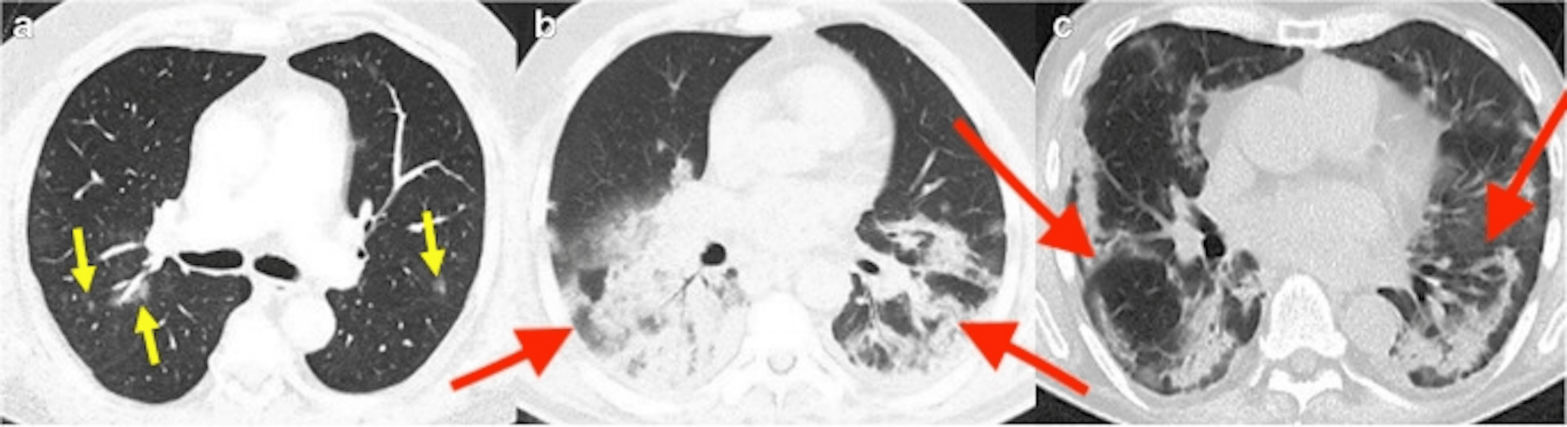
Representative CT findings in COVID-19 pneumonia CT: computed tomography Transverse CT images show (A) ill-defined ground-glass nodules bilaterally (yellow arrows), (B) extensive ill-defined consolidations with positive air bronchograms in bilateral lower lobes (red arrows), and (C) consolidations in the lung periphery sparing the subpleural space (red arrows). Image adapted and reproduced from Afshar-Oromieh et al. [2] under the CC BY 4.0 license
